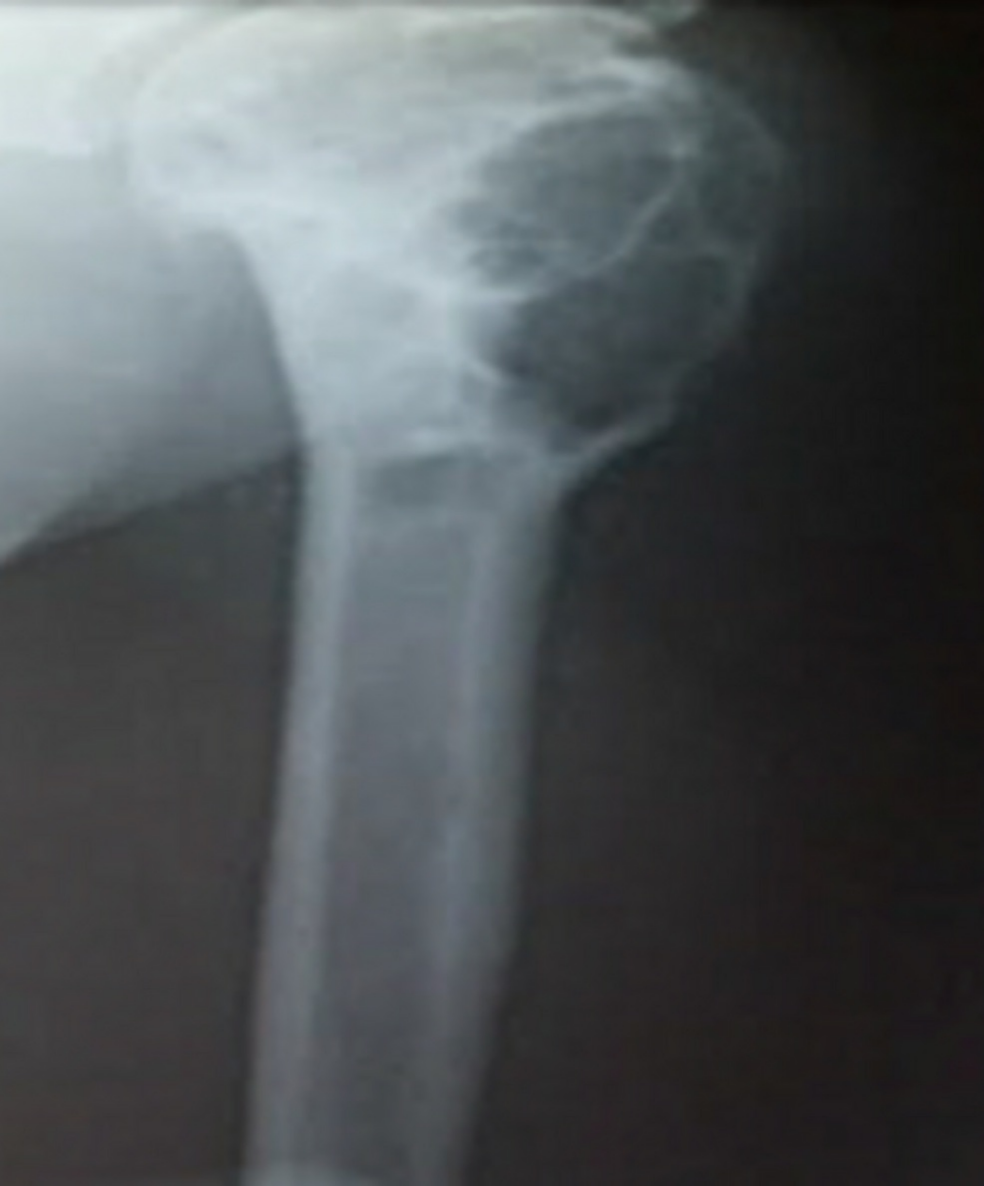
Preoperative X-ray showing recurrence for the first time following previous curettage and grafting (2003)
